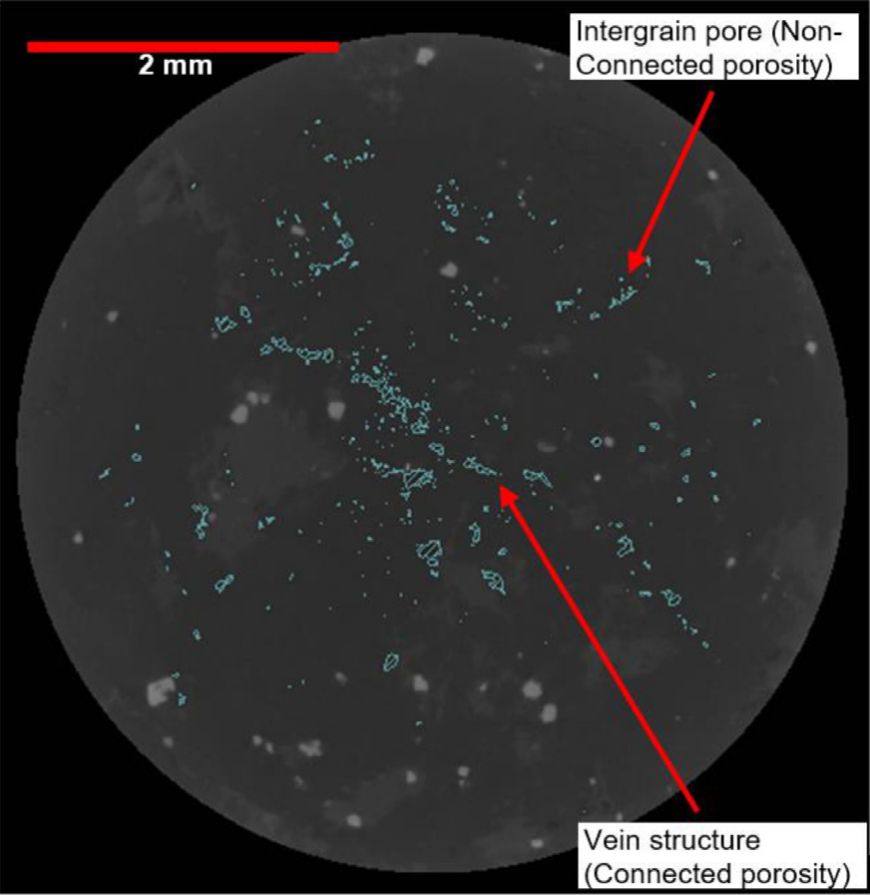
X-Ray micro-tomography 2D slice with voids highlighted.
